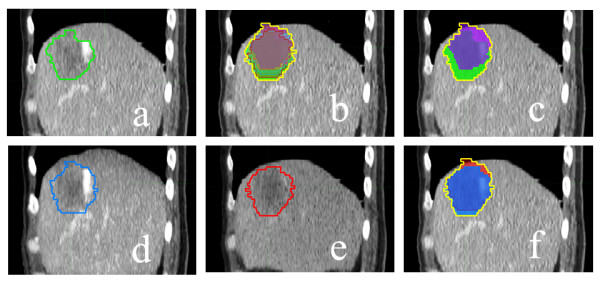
Panel (a) shows the GTV (green contour) for patient 13; Delineation of ITV base on ITV_AllPhases_, ITV_2Phase_, ITV_MIP_, ITV_MinIP _and ITV_2M _are shown in panels (b), (c), (d), (e) and (f), respectively. ITV_MIP _and ITV_MinIP _coutours are as they appear on the intensity projection data set; all others are registered to the 0% phase of the 4D CT data set.
